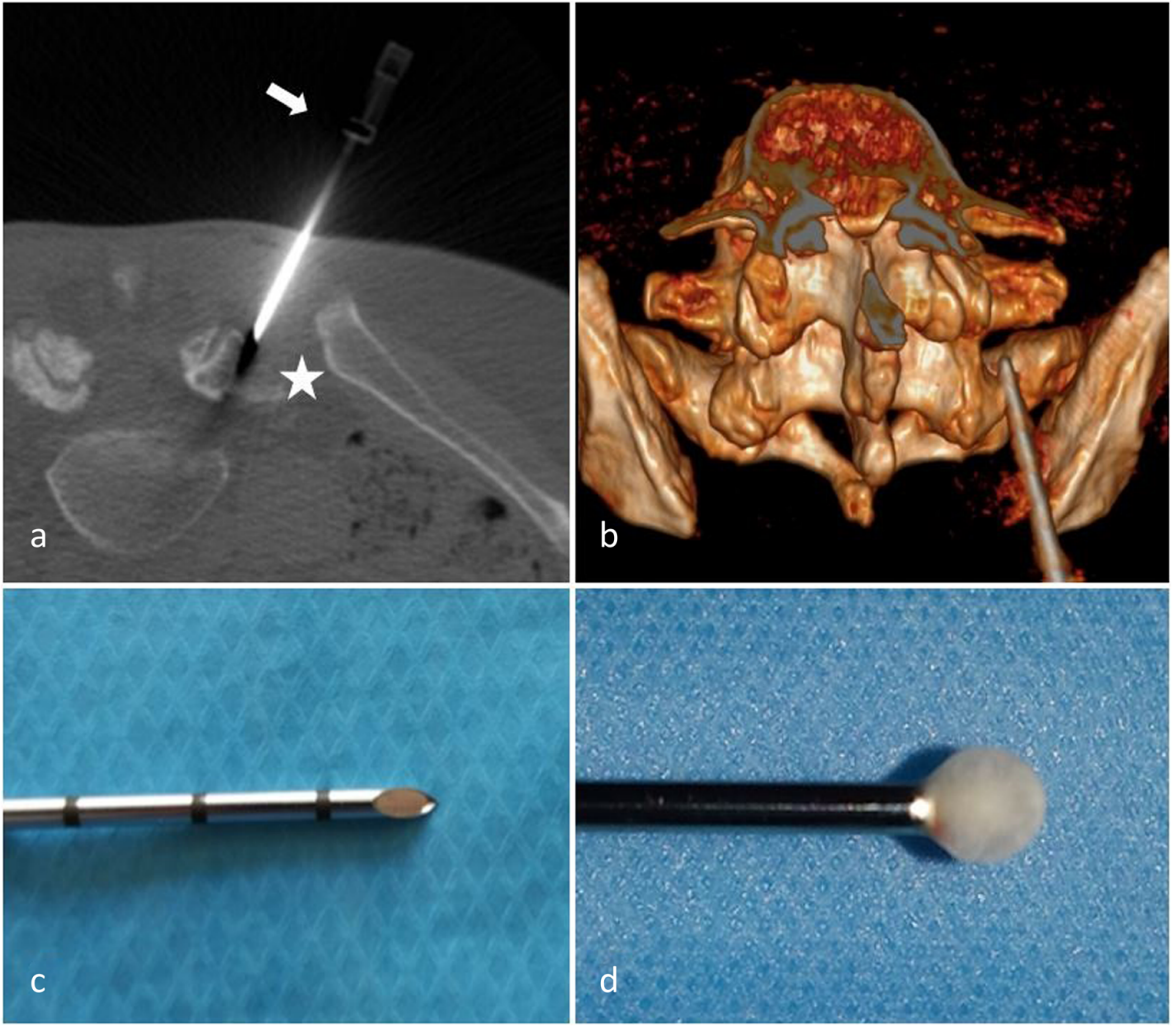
Facet joint cryoneurolysis. CT-guided cryoablation at the right L5-S1 level. a: Anatomically accurate cryoprobe (white arrow) placement with the target midway between the upper end and middle of the ala of the sacrum (white star). b: 3D reconstruction showing the same cryoprobe placement. Cryoprobe needle tip (c): ice-ball formation at the cryoprobe tip (Joule–Thompson effect) (d)
